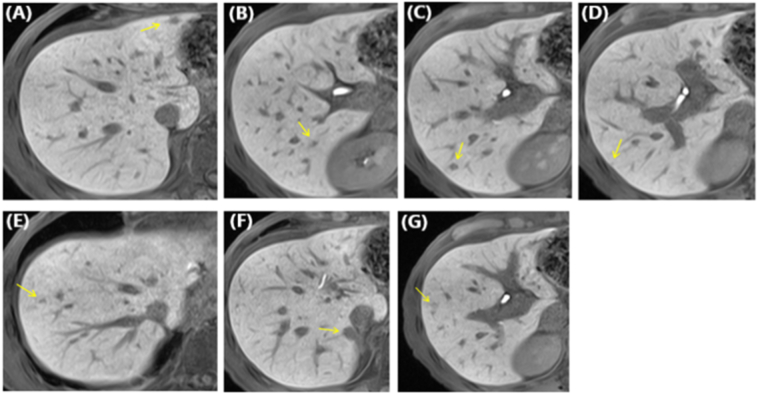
Ethoxybenzyl-enhanced magnetic resonance imaging findings following chemotherapy. The liver tumors (yellow arrows) in segments 3 (A), 5 (G), 6 (B, C, D), 7 (F), and 8 (E) are reduced. No new lesions are noted. (For interpretation of the references to colour in this figure legend, the reader is referred to the web version of this article.)
